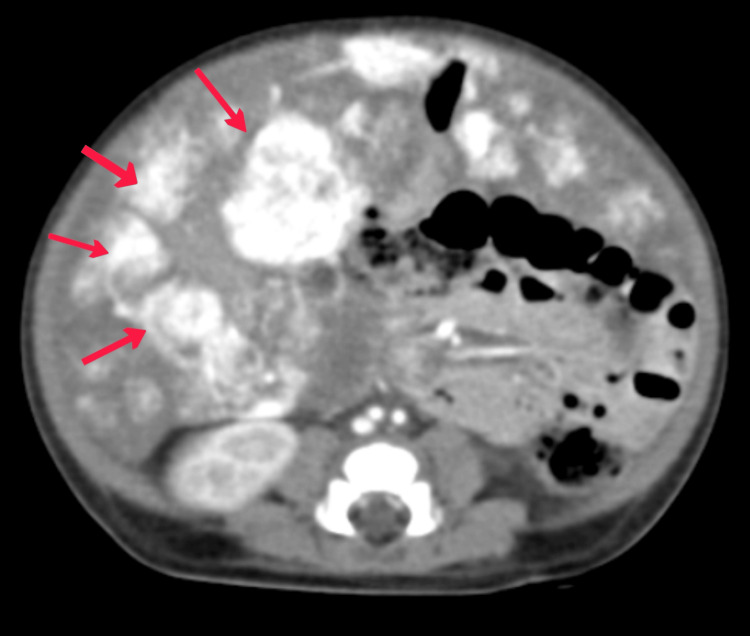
CT scan shows multiple large multilobulated mass CT: Computed tomography
